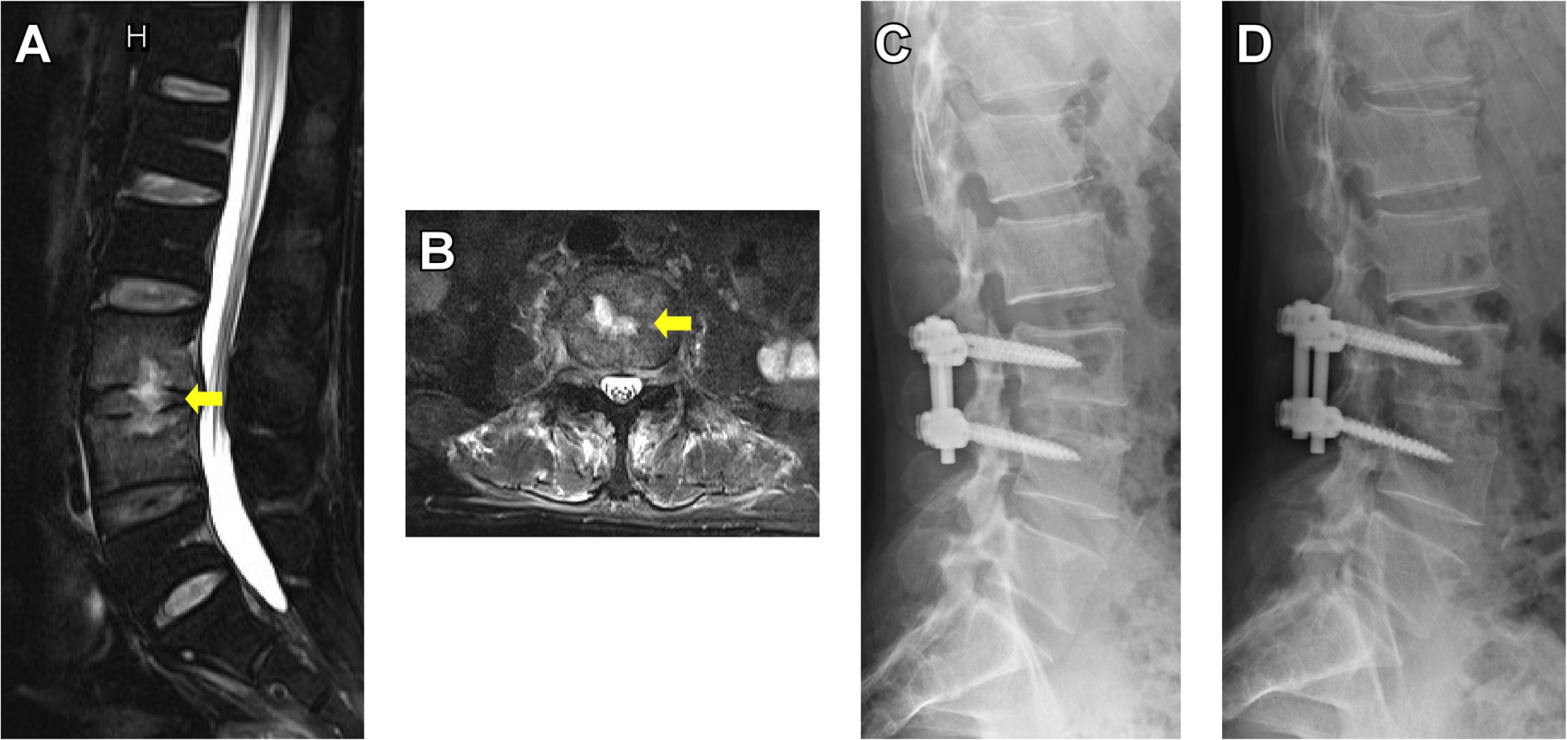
Pre- and post-operative radiological images of a 54-year-old male with intractable low back pain and limited range of motion of waist. a, at admission, endplate erosion and abscess (yellow arrow) at L3/4 intervertebral were disc seen on T2-weighted MRI. b, the abscess (yellow arrow) localized at the center of the disc. c, postoperative X-ray filming at the 1-month follow up. d, X-ray filming in 2 years after treatment
